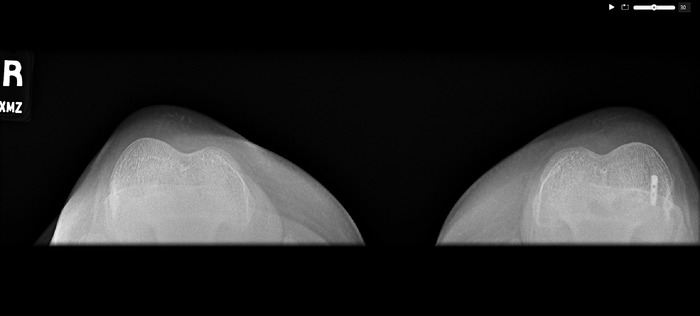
Skyline radiograph of both knees shows patellar aplasia.
